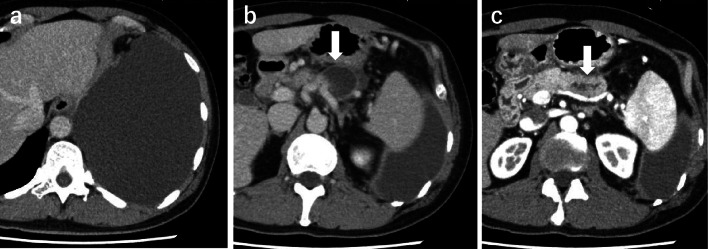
Findings of computed tomography (CT). CT reveals left-sided pleural effusion (a). Pancreatic pleural effusion was diagnosed on pleural puncture, based on the high amylase content (36,854 IU/L) in the pleural fluid. b CT shows a pseudocyst (arrow). c Dilation of the main pancreatic duct (arrow) in the pancreatic tail. No tumor can be observed in the pancreas
